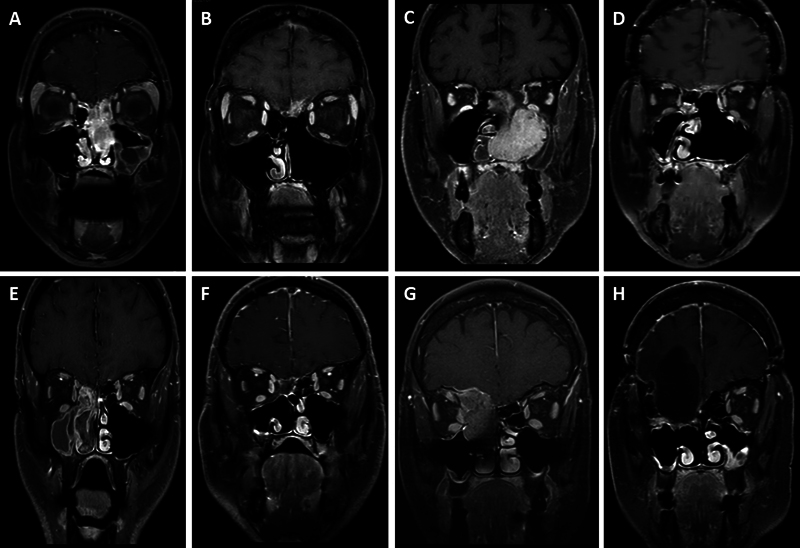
Radiological imaging of sinonasal cancers. This figure demonstrates four cases with a pre- and postoperative contrast enhanced T1 MRI in the coronal plane. (
